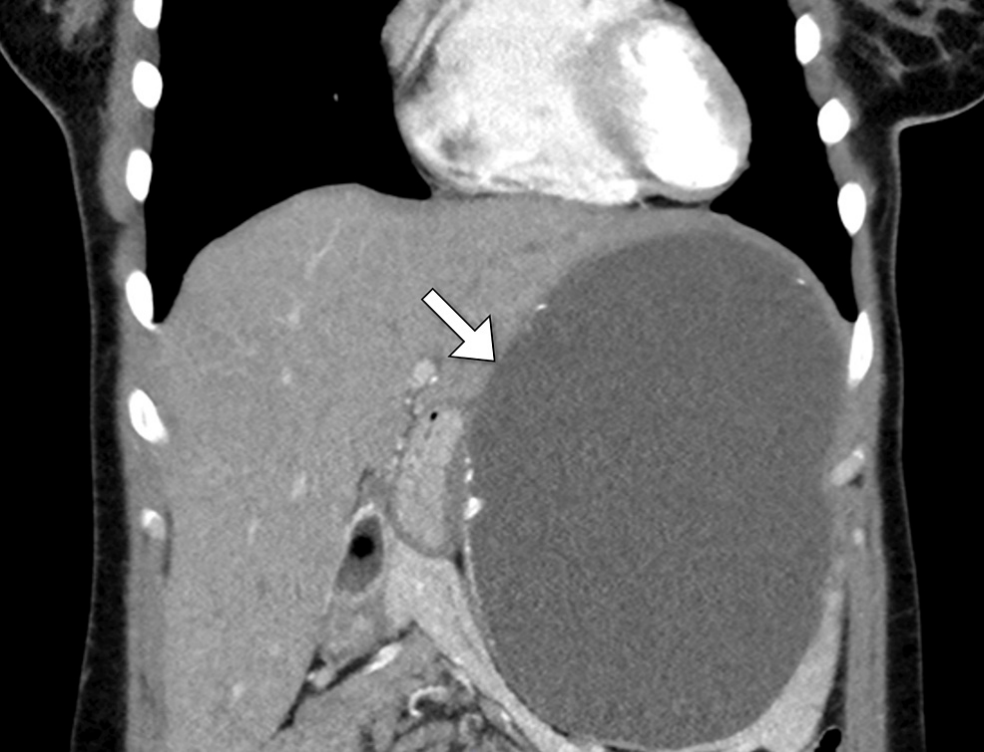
Coronal CT image of the abdomen showing a cystic lesion (arrow) within the spleen with thin marginal calcification. CT: computed tomography
